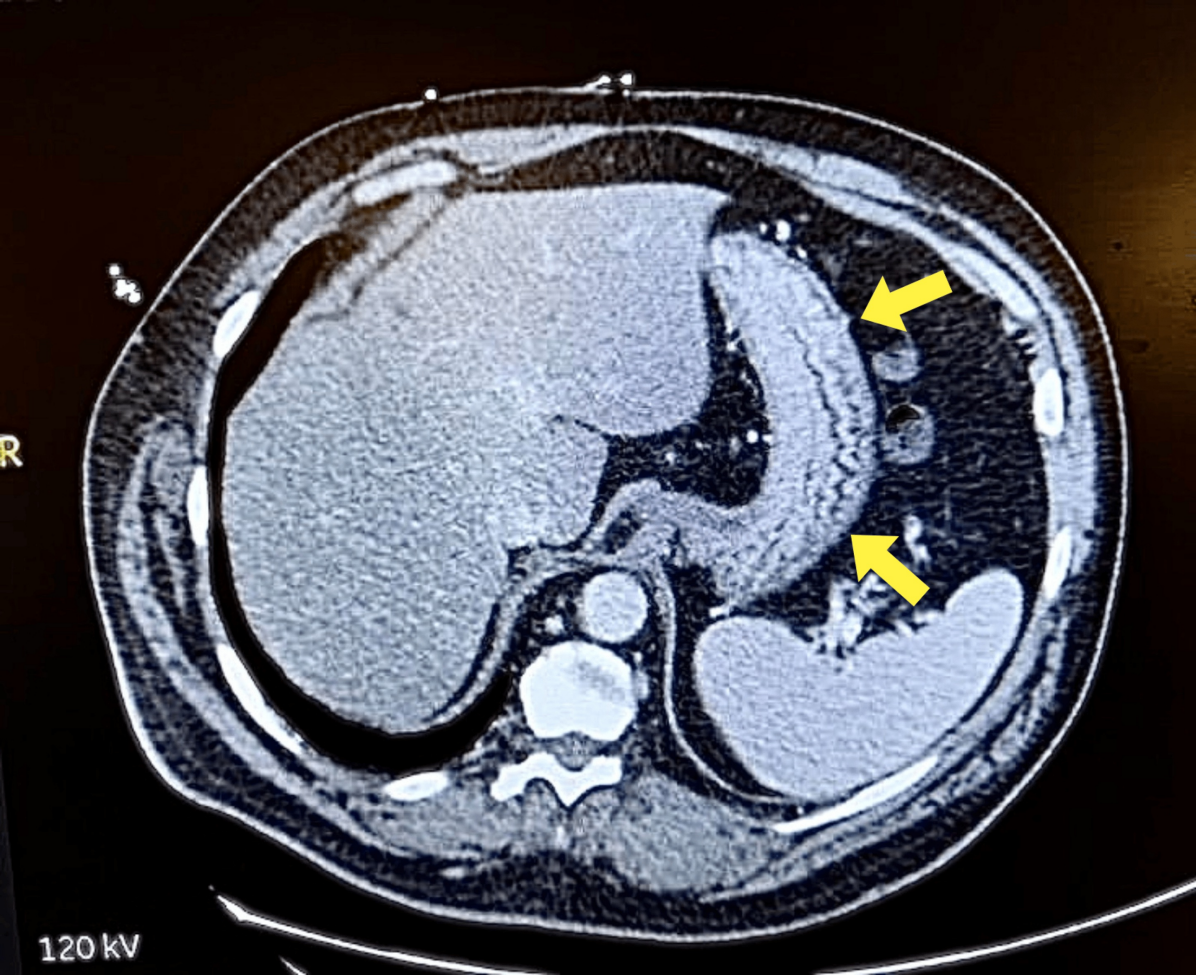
Transverse contrast CT abdomen showing gastric dilation
